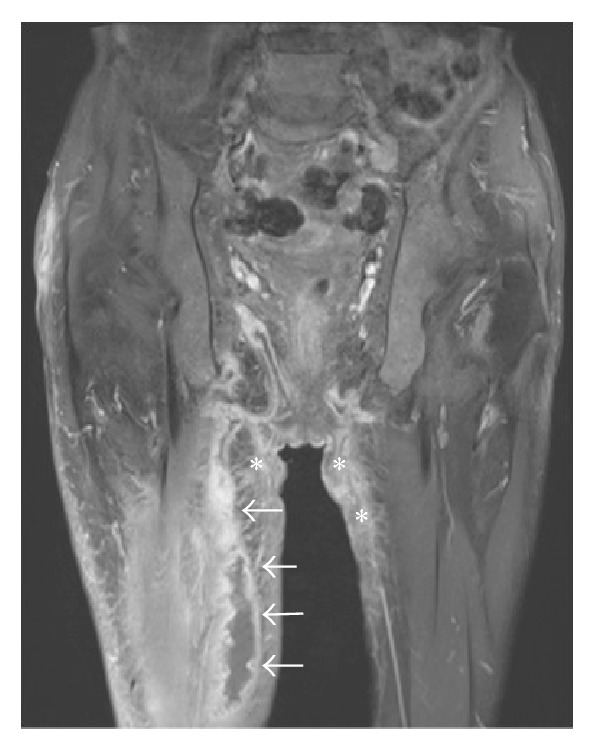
Coronal T1-weighted fat-suppressed image postcontrast shows inferior extension of the rim-enhancing abscess along the medial aspect of the right thigh (white arrows). Note: associated bilateral dermal induration best seen in the perineum with abnormal enhancement (∗).
